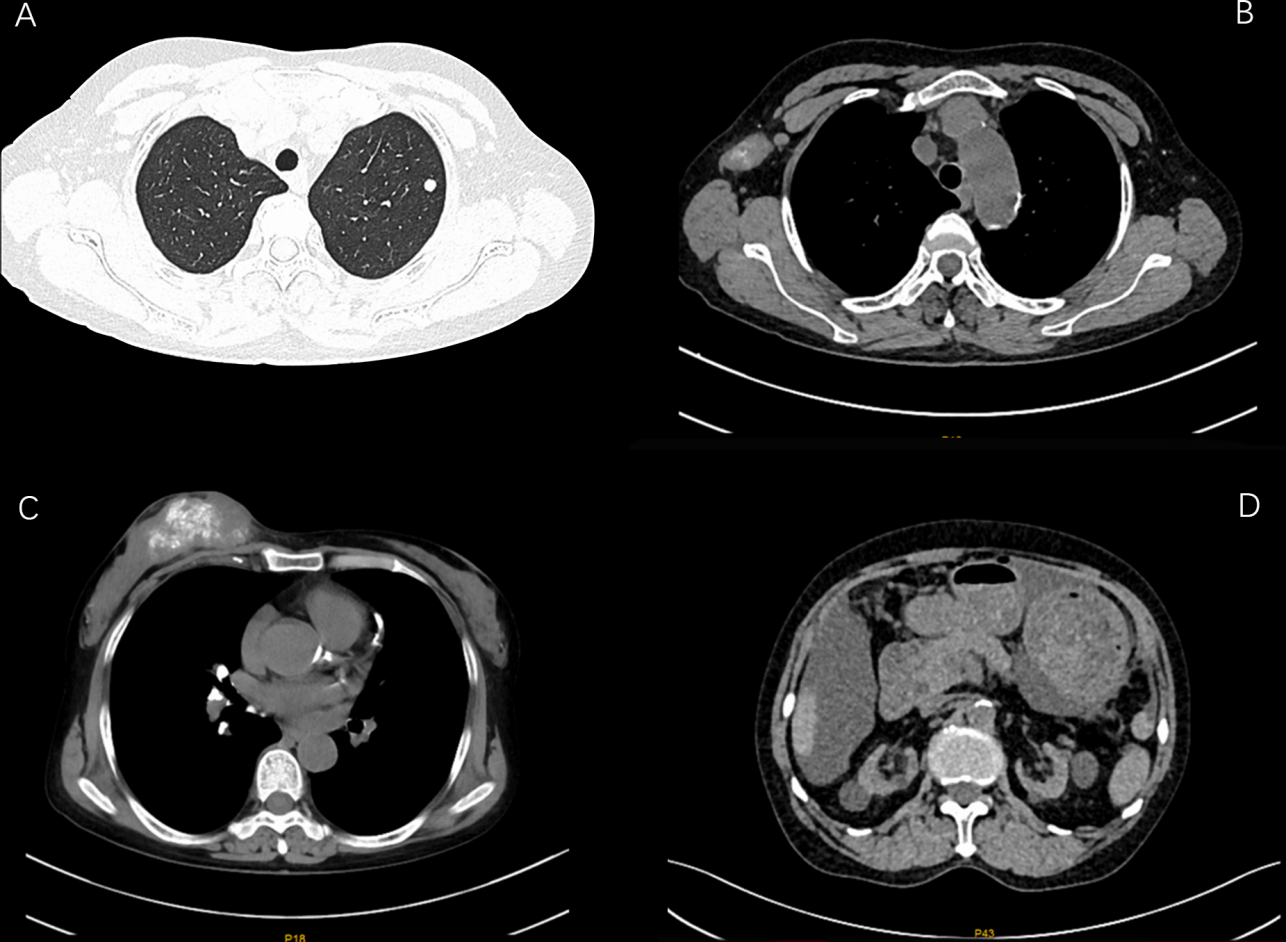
CT images of patient before anti -tumor treatment (A: lung nodules B: lymph nodes in the armpit C: massive soft tissue density with multiple calcifications in the right breast D: interval).
